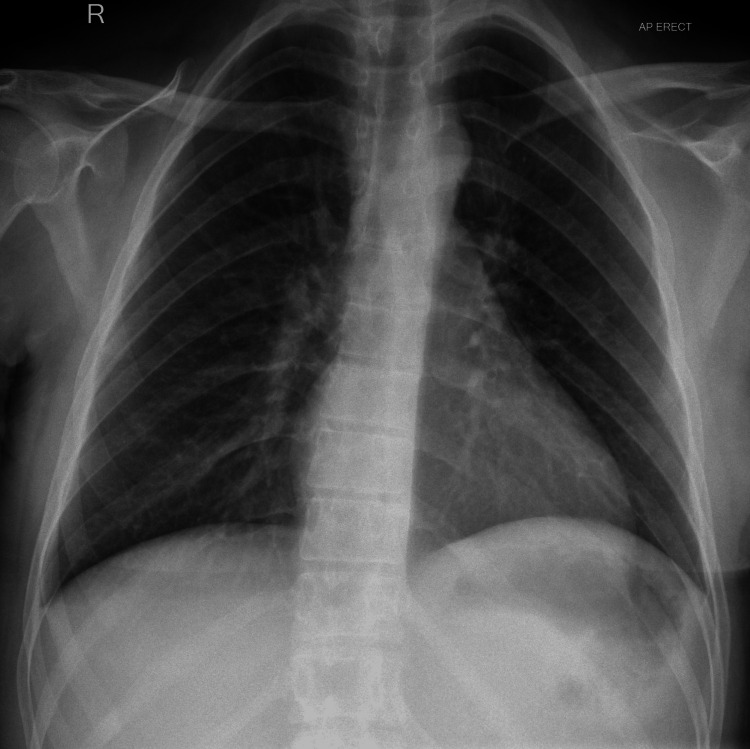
Chest X-ray before discharge during a previous admission.
